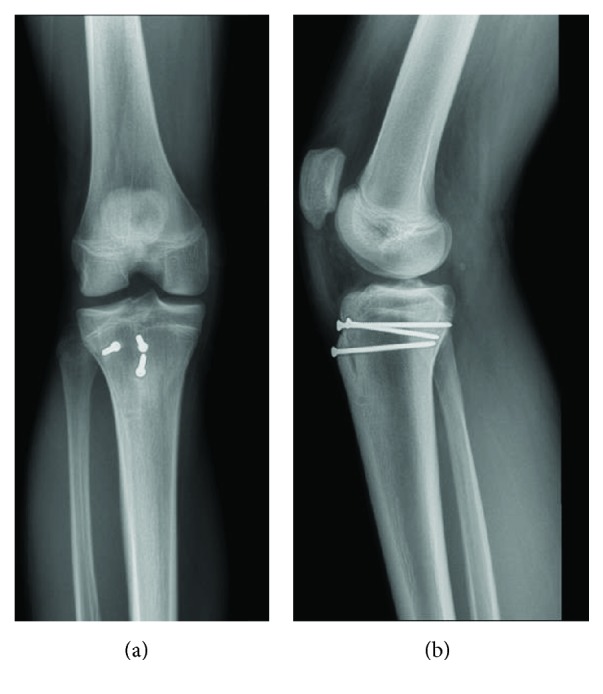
Anterior-Posterior (a) and lateral (b) X-rays 6 months following operative management demonstrates well-positioned screws with callus formation at the site of injury.
